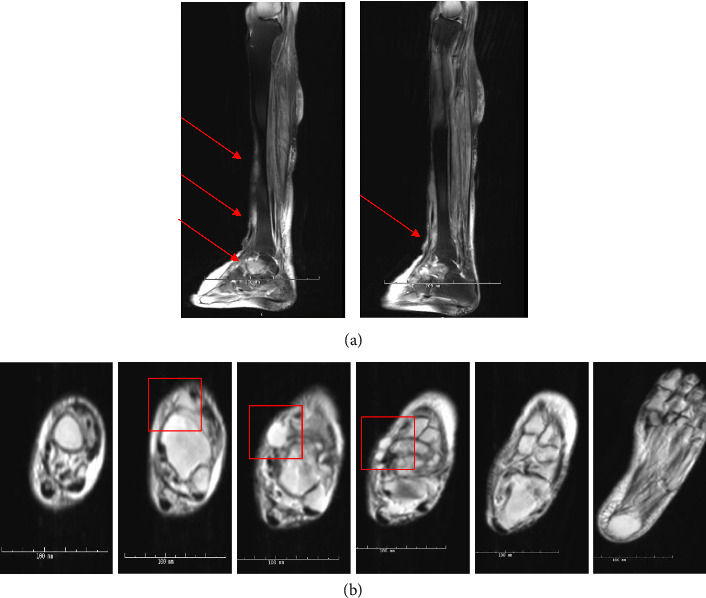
Magnetic resonance imaging of the lower left limb at index admission. (a) Sagittal evaluation of the lower left limb with associated high density opaque regions (red arrows). (b) Evaluation of the lower left limb at index admission across caudal axial series with associated high density opaque regions (red arrows).
